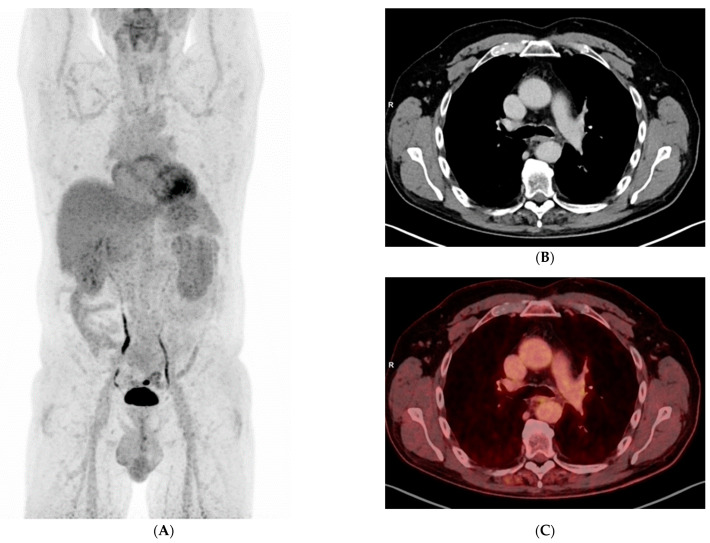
(A) MIP FDG-PET showing complete resolution of all the prior FDG-avid lesions, (B) transaxial CT image showing resolution of the prior tumor atelectasis complex, (C) same as (B) fused with FDG-PET.
